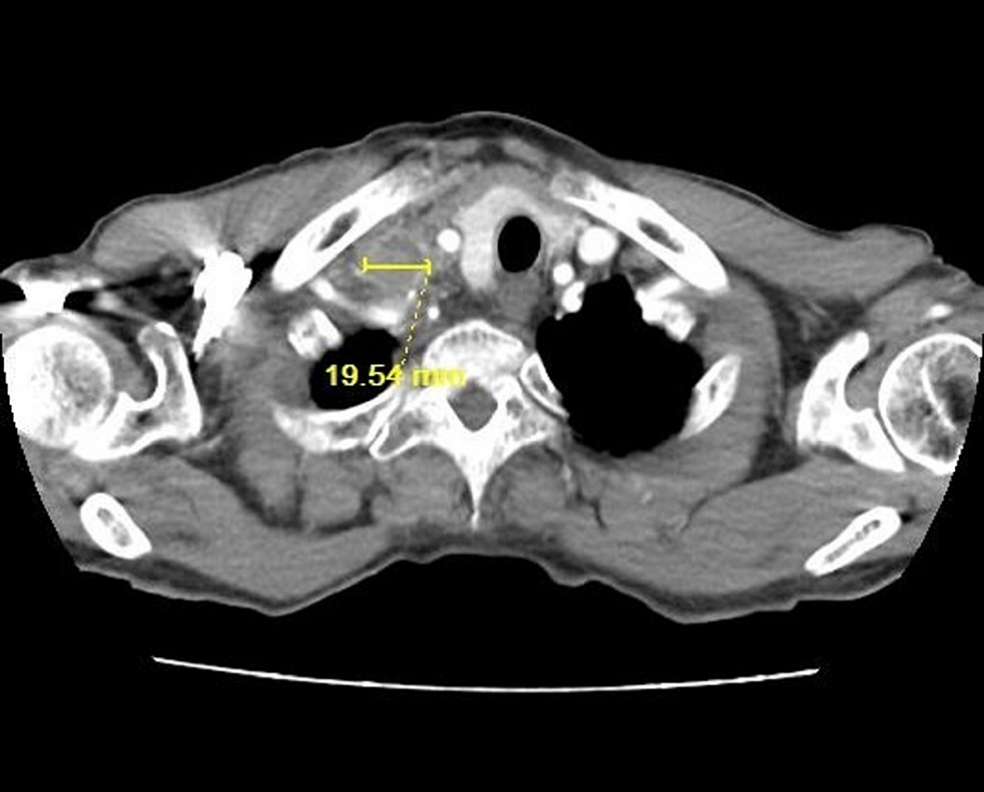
CT chest soft tissue window showing right subclavian lymph nodes, with the largest measuring 19.54 mm, on 06/12/2017
